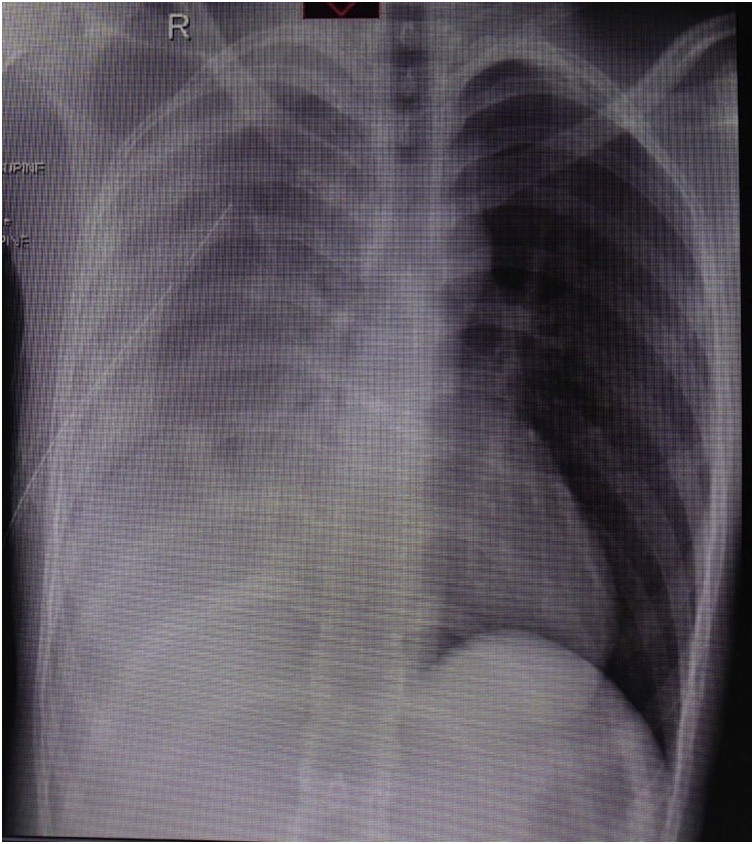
Chest x-ray post thoracostomy tube insertion showed right pneumothorax and opacity.
